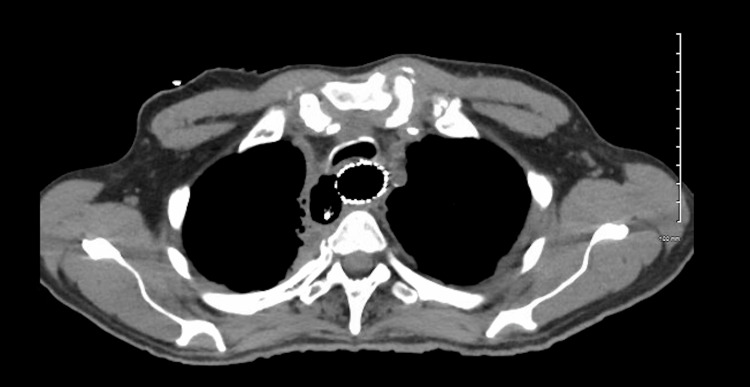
Computed tomographic images status-post esophageal stent placement
